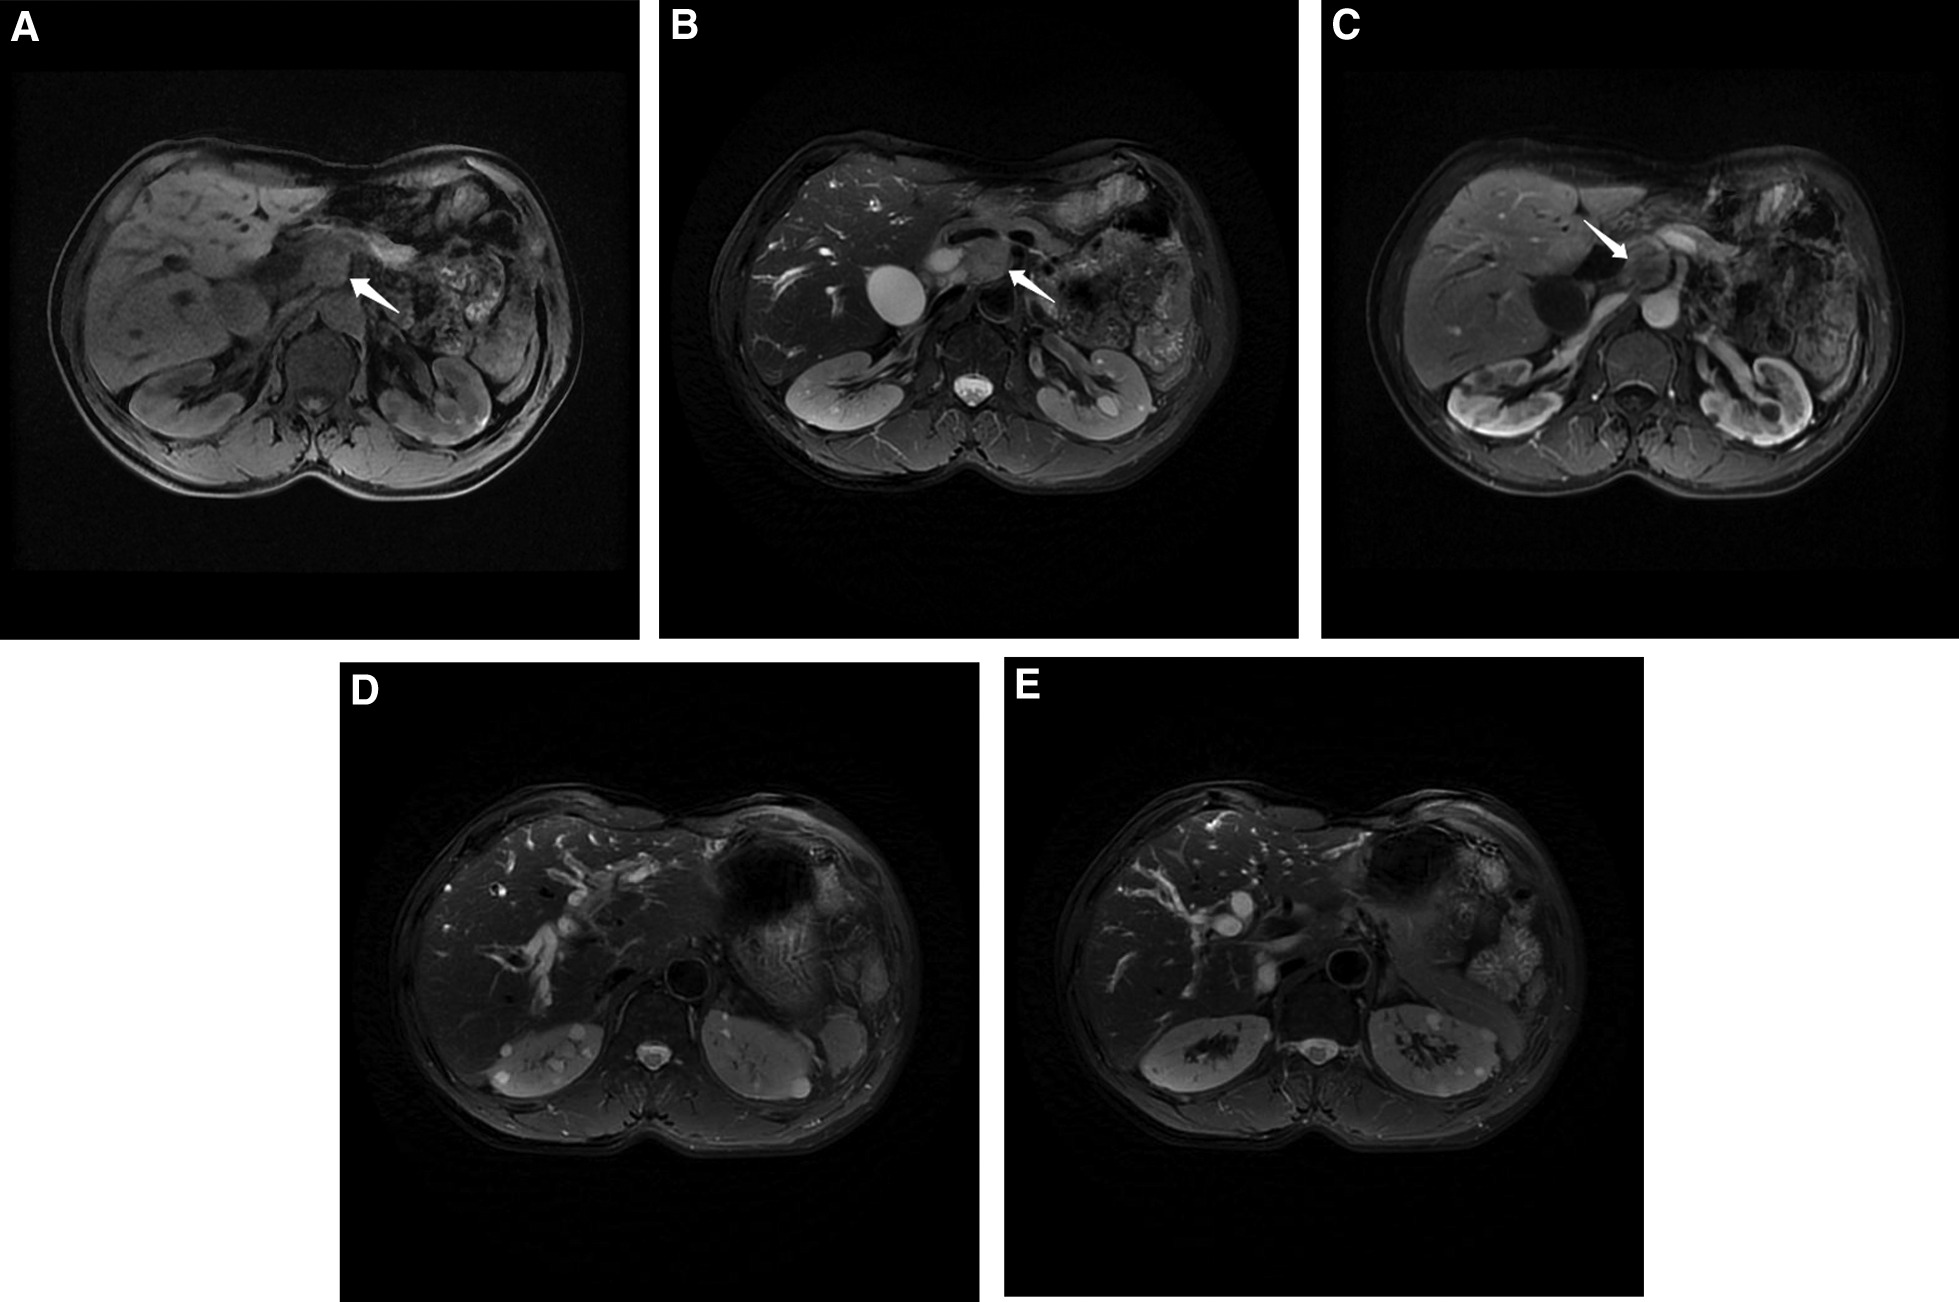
Enhanced magnetic resonance imaging. a T1WI showed low signal intensity of a pancreatic head mass. b On T2WI, the mass of the head of the pancreas shows iso-signal intensity, and the common bile duct, abdominal trunk, and other branches were surrounded. c MR-enhanced pancreatic head mass showed uneven enhancement. d Intrahepatic bile duct dilatation. e No significant swelling or distal pancreatic duct widening was observed in the body and tail of the pancreas
